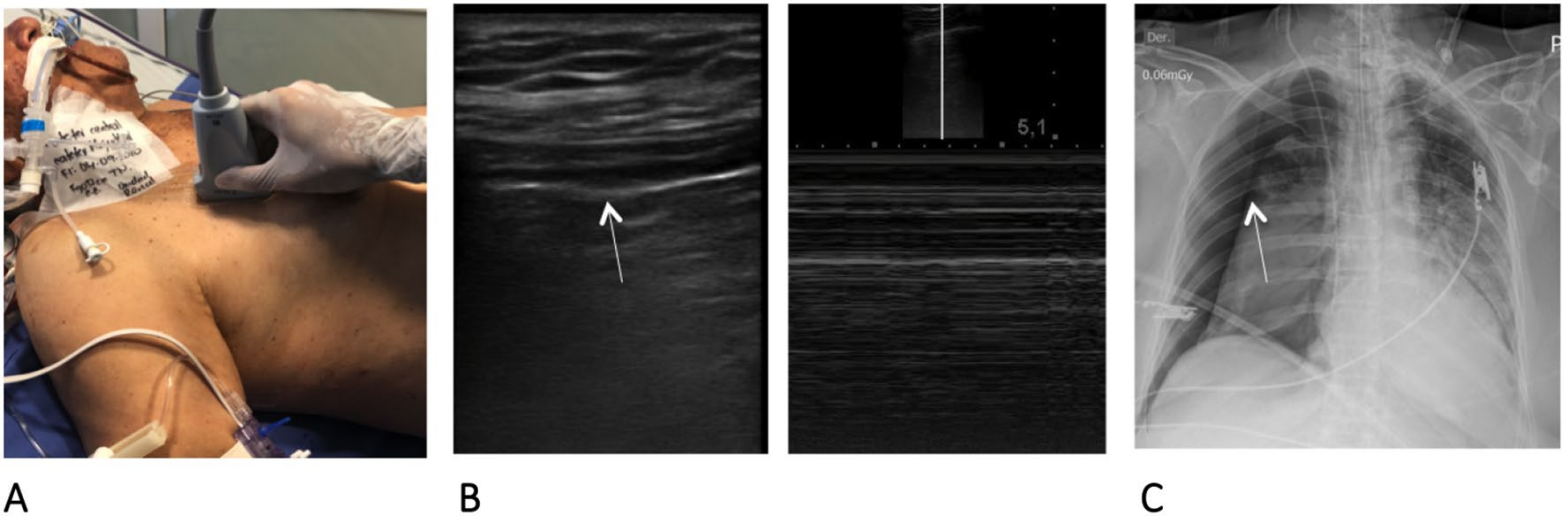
A Evaluation of the presence of pneumothorax with a high-frequency linear transducer (13 MHz) B. Pneumothorax was diagnosed due to the absence of pleural sliding (arrow) and the barcode sign in M-mode C. Chest radiograph confirming the presence of pneumothorax (arrow)
